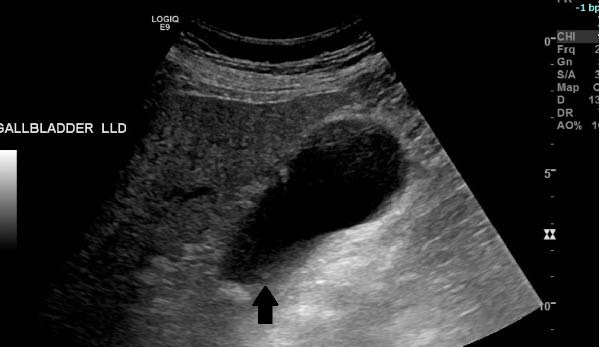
Right upper quadrant ultrasonography showing a distended gallbladder with cholelithiasis (arrow).
